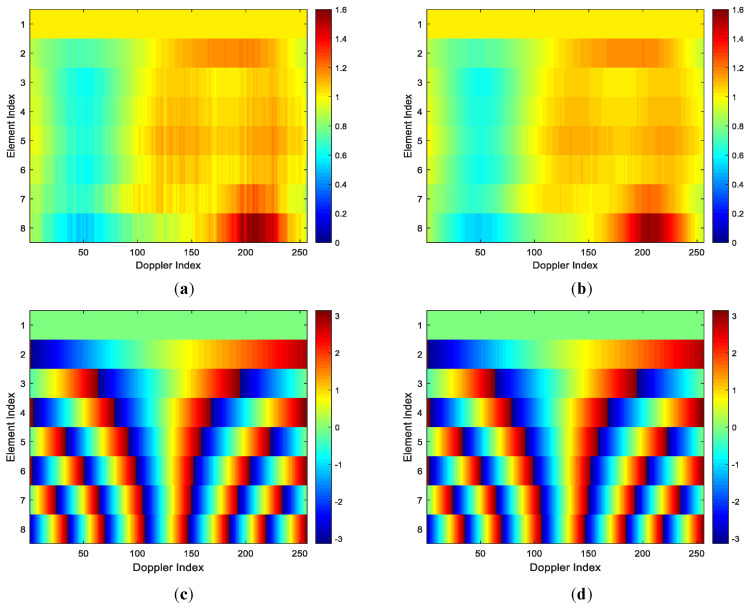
Steering vector estimation results of all Doppler bins. (a) Amplitudes of estimated steering vectors; (b) Amplitudes of true steering vectors; (c) Interferometry phases of estimated steering vectors; (d) Interferometry phases of true steering vectors.
